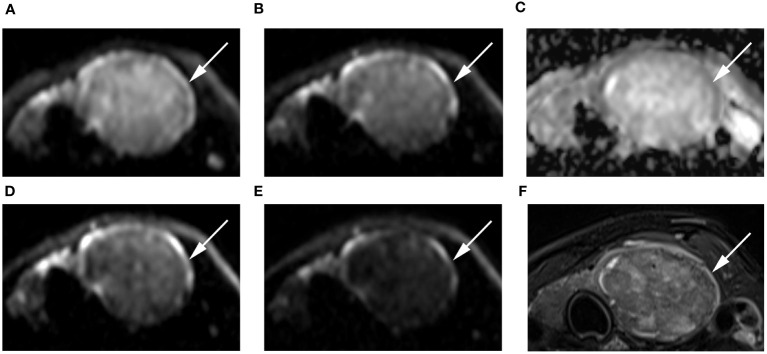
Images of a 55-year-old female with left lobe nodular goiters (arrow): DWI image with a b-value of 500 s/mm^2^
